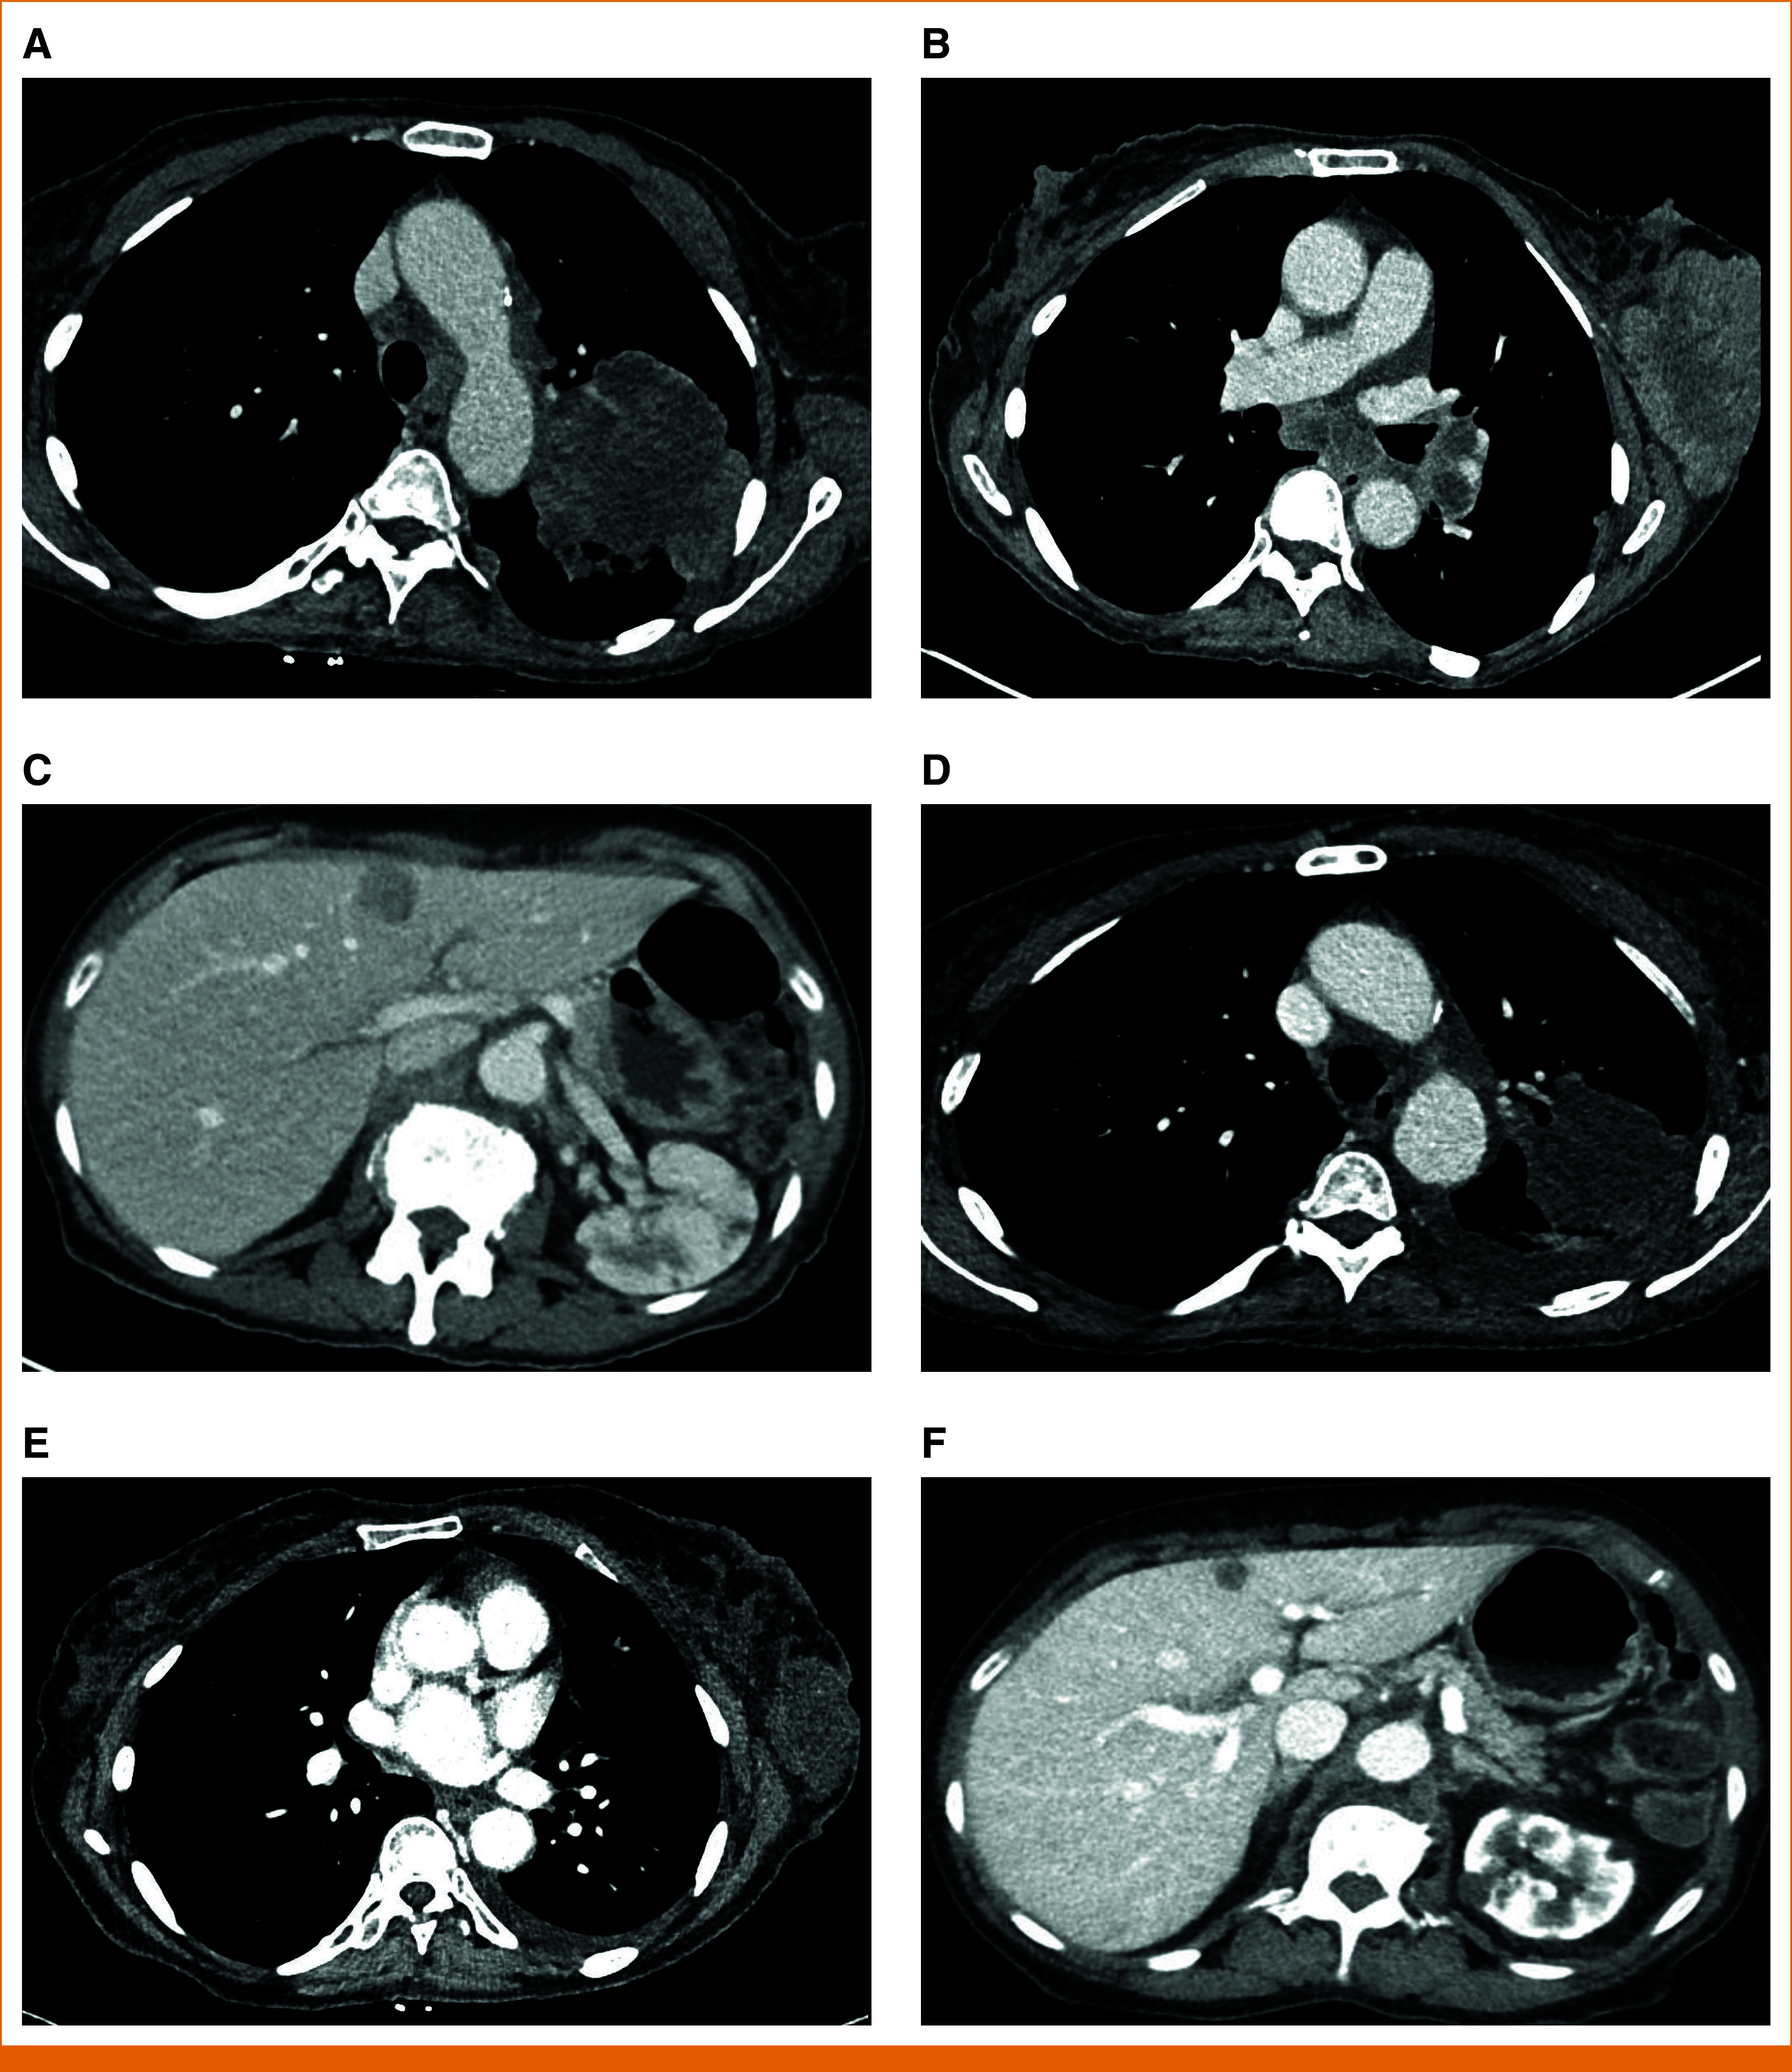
(A) Axial image from chest CT with contrast shows a lobulated heterogeneously enhancing mass in the left upper lobe measuring up to 7.9 cm. (B) Necrotic subcarinal, left perihilar lymphadenopathy (arrows). A large lobulated heterogeneous left breast mass measuring up to 9.4 cm (partially imaged) is also noted. (C) CT of abdomen with contrast performed before treatment shows 22 × 20 mm left hepatic mass. (D) Axial image from chest CT with contrast performed 3 months after treatment shows the interval decrease in size of left upper lobe mass and mediastinal and left perihilar lymphadenopathy. (E) Axial image from chest and left breast mass CT with contrast performed 3 months after treatment with increased areas of low attenuation within. (F) CT of abdomen with contrast performed 3 months after treatment shows the interval decrease in size of hepatic metastatic lesion to 11 × 12 mm. CT, computed tomography.
